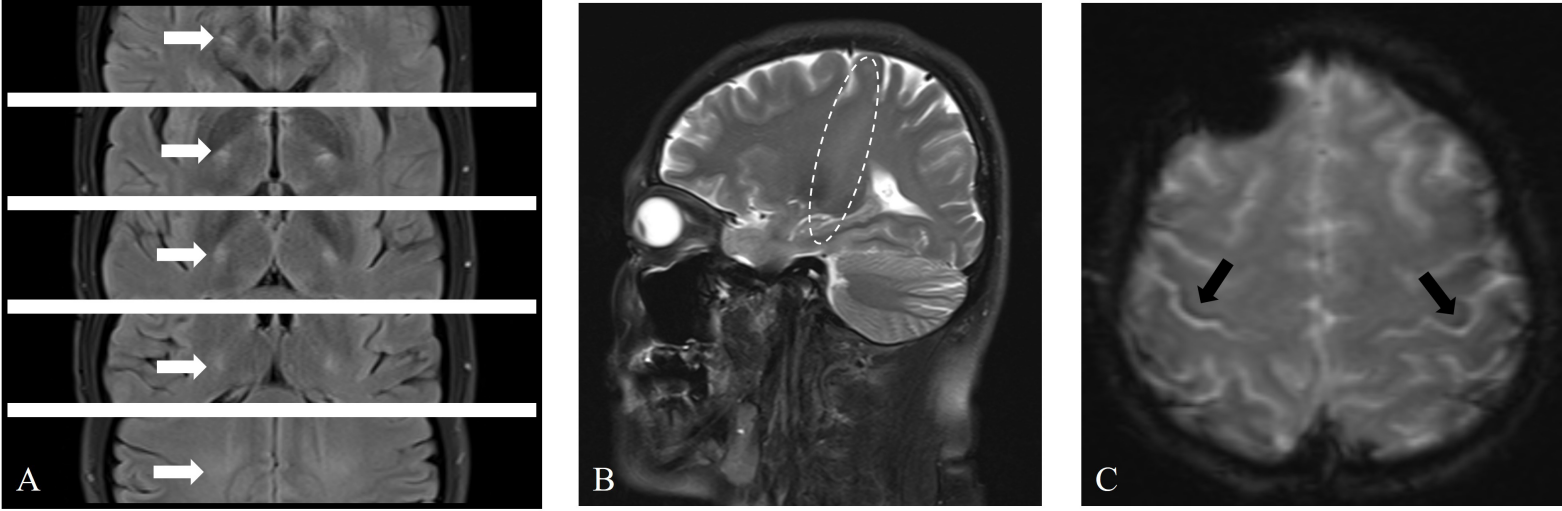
Brain magnetic resonance imaging (MRI) revealed abnormal signals
